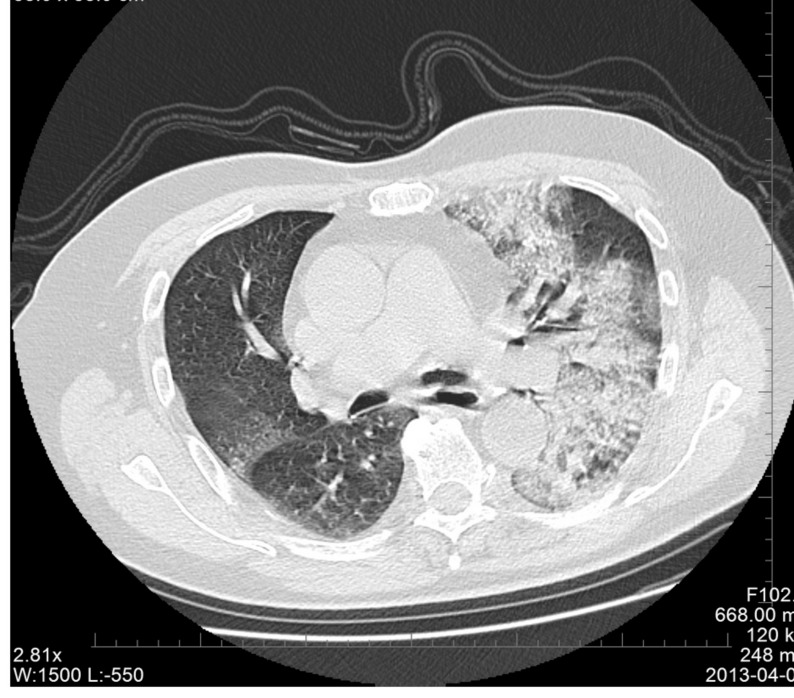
On April 7^th^ 2013, the chest computed tomography scan revealed the left lower lung with patchy inflammation.
